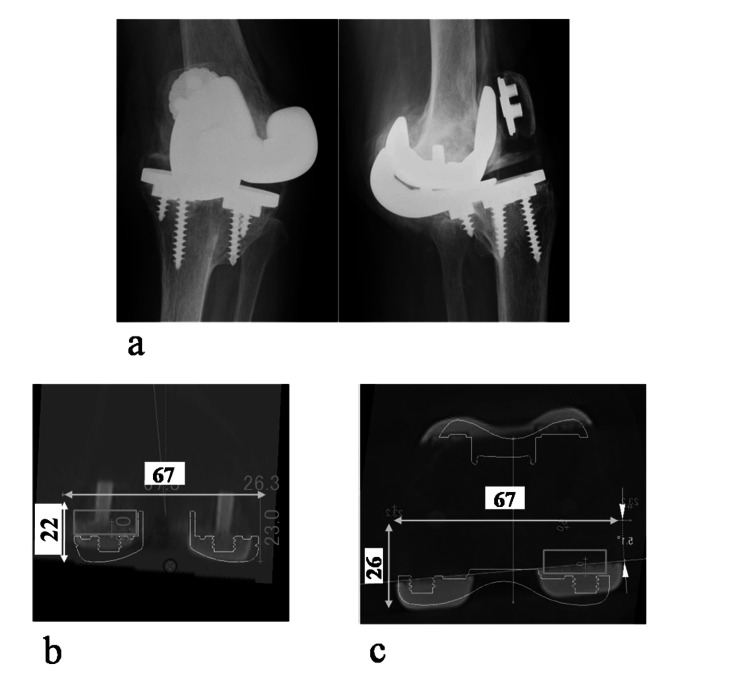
Preoperative planning for revision TKA using 3D preoperative planning software (Athena). (a) Radiographic image of revision case. (b) Coronal view: restoration of the distal femoral joint line (ML, 67 mm x 0.33 mm = 22 mm from the ME). (c) Axial view: restoration of the posterior femoral joint line (ML, 67 mm x 0.39 mm = 26 mm from the ME).
